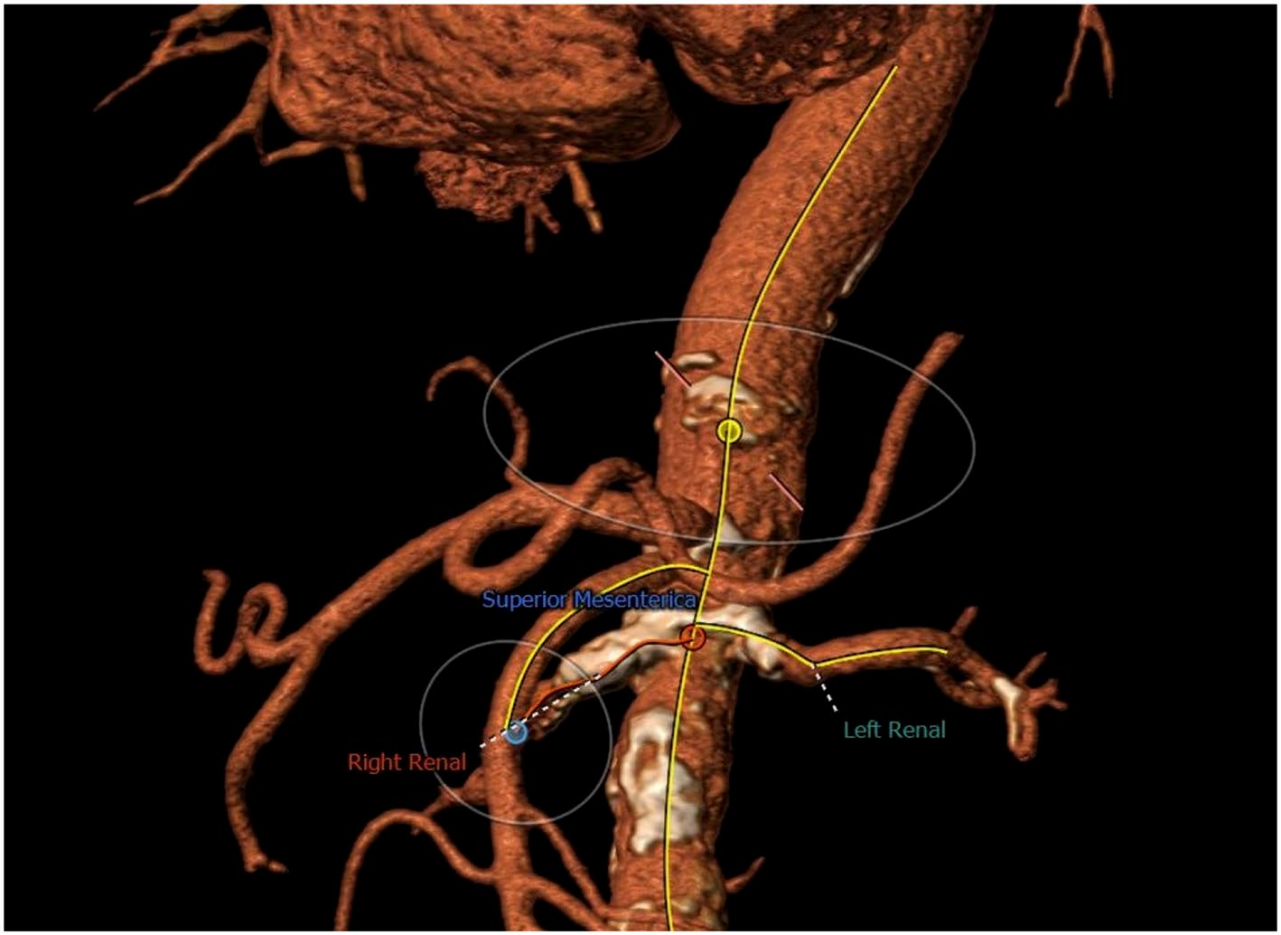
3D CT scan reconstruction of patients´ individual anatomy showing coral reef aorta
